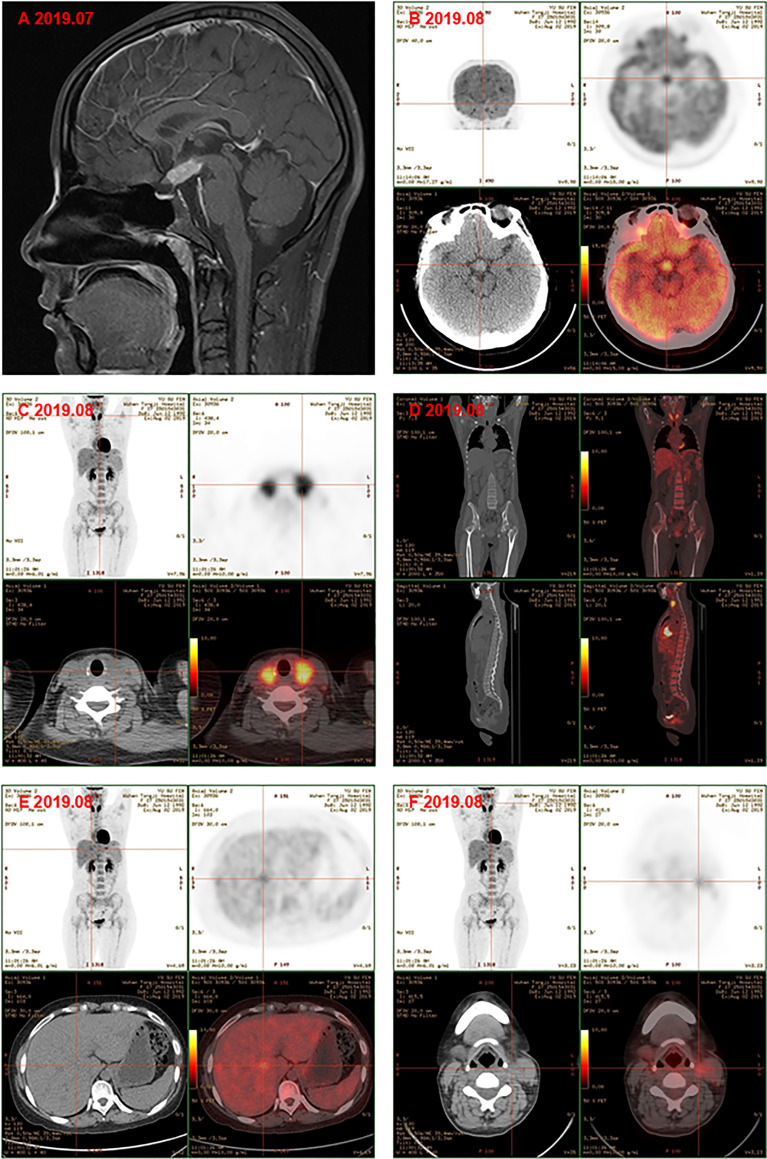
(A) Magnetic resonance image of pituitary gland showd the nodular thickening and enhancement of the hyphothalamus, and the pituitary itself became thinner. PET-CT showed the metabolism of hypothalamus was higher (B); the volume of bilobe thyroid and metabolism increased (C); multiple lymph nodes icreased and metabolism increased, bone marrow metabolism slightly increased (D); multiple slightly low density shadows in the liver (E); bilateral tonsil metabolism increased (F) before chemotherapy.
